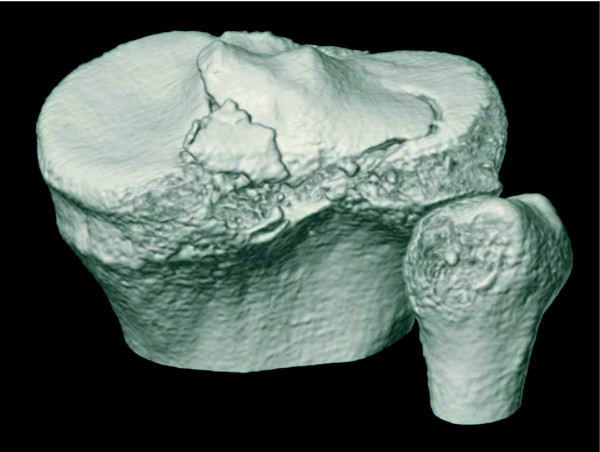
Computed tomography scans showing three-dimensional reconstruction views of the knee: the displaced fragments of the posterior intercondylar eminence and the lateral tibial condyle can be observed.
